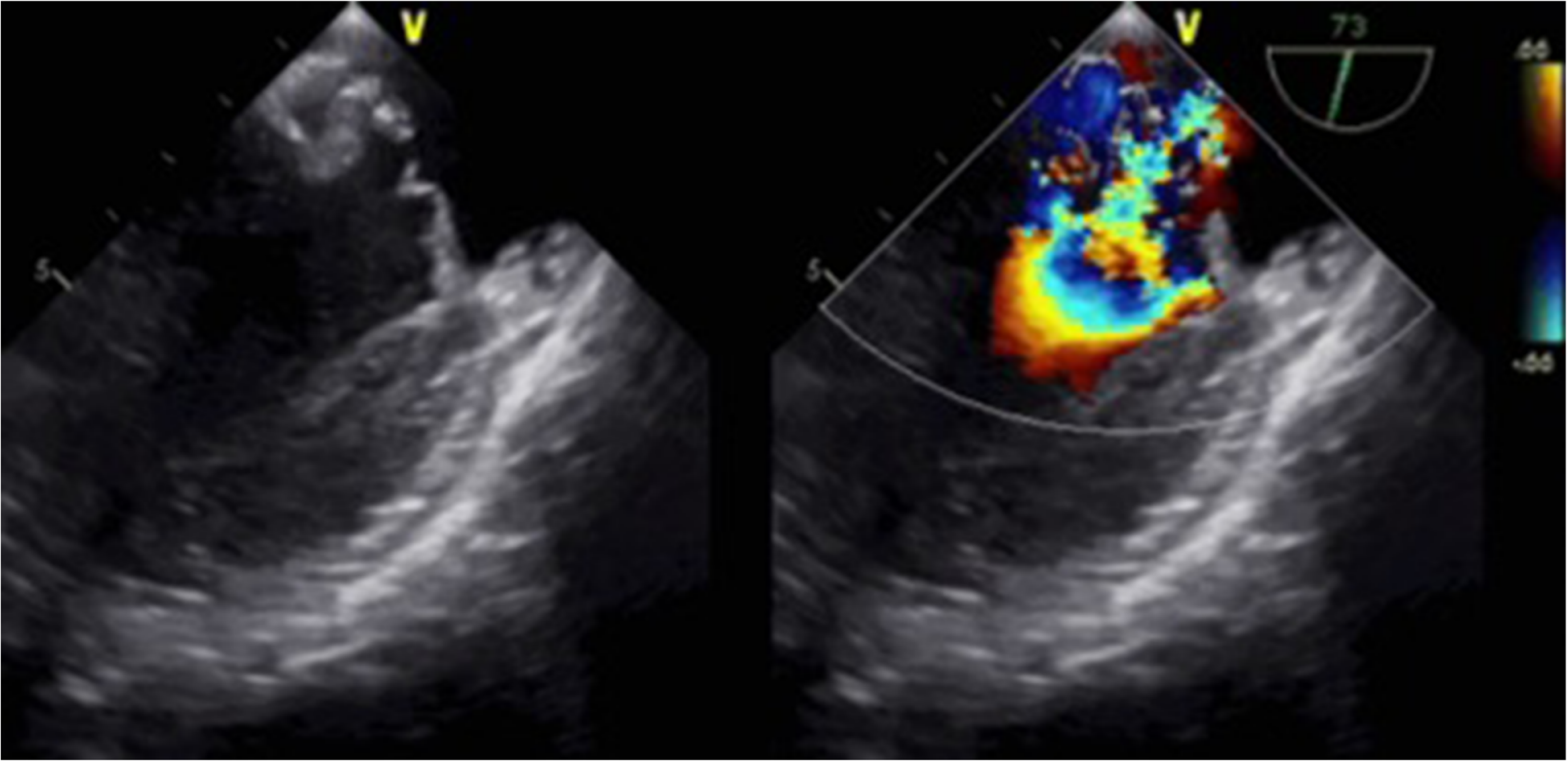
Transesophageal echocardiography showed acute mitral regurgitation and mitral valve tendon rupture
